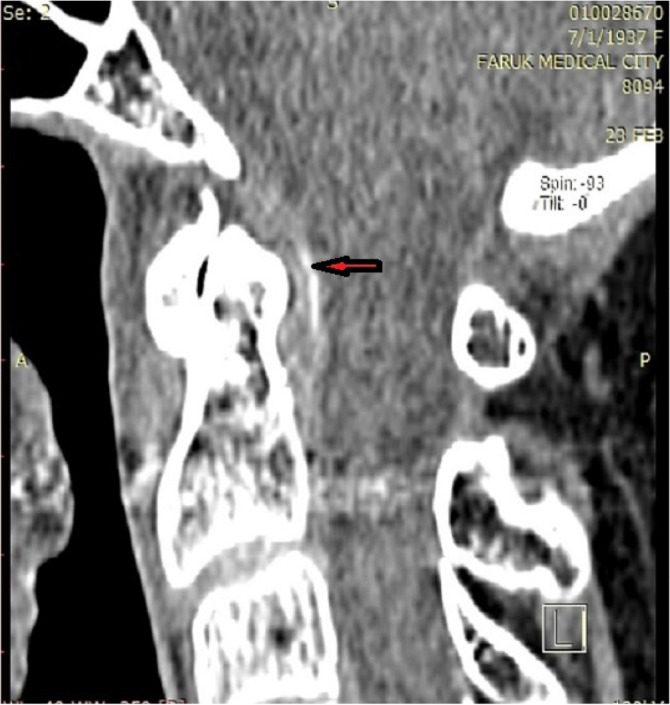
Cervical CT: linear calcification posterior to the dens (sagittal).
